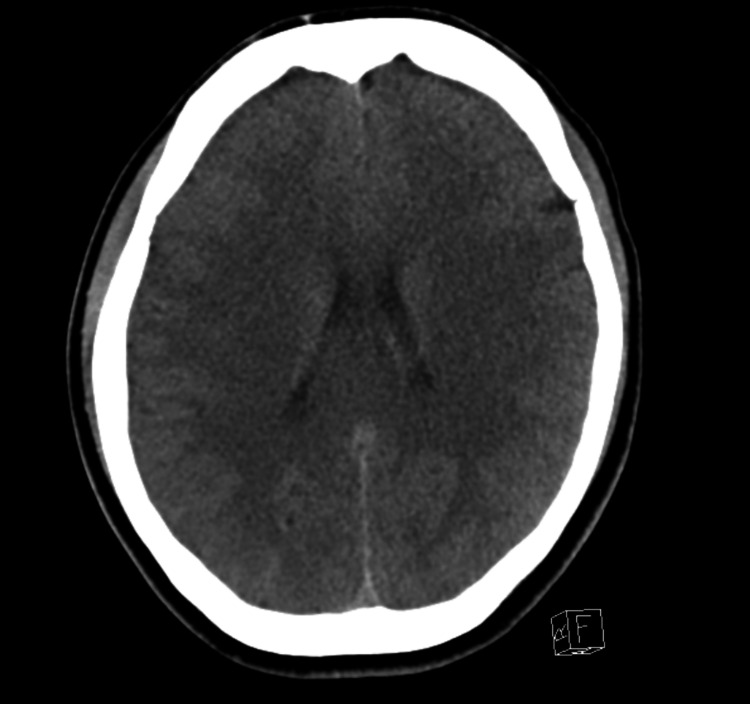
CT scan of the head was negative for bleeding or infarction
